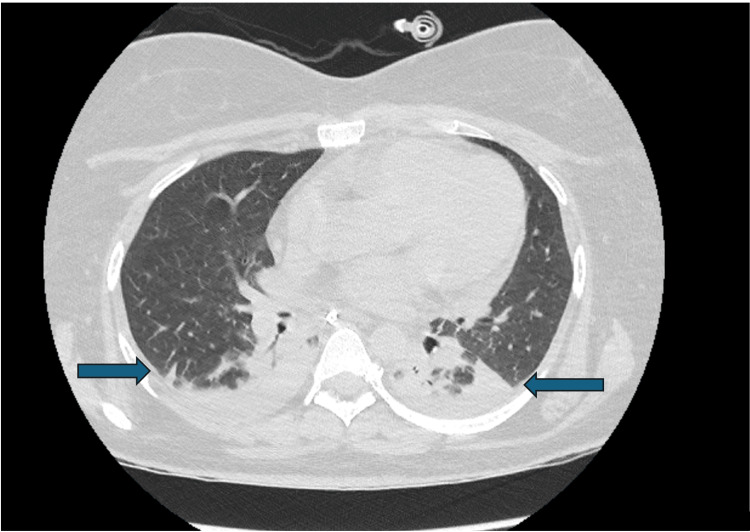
CT scan of the chest. CT scan of the chest demonstrating aspiration pneumonitis, bilateral lower lobe collapse, and small pleural effusions (indicated by arrows). The CT of the neck was performed but showed no significant findings.
